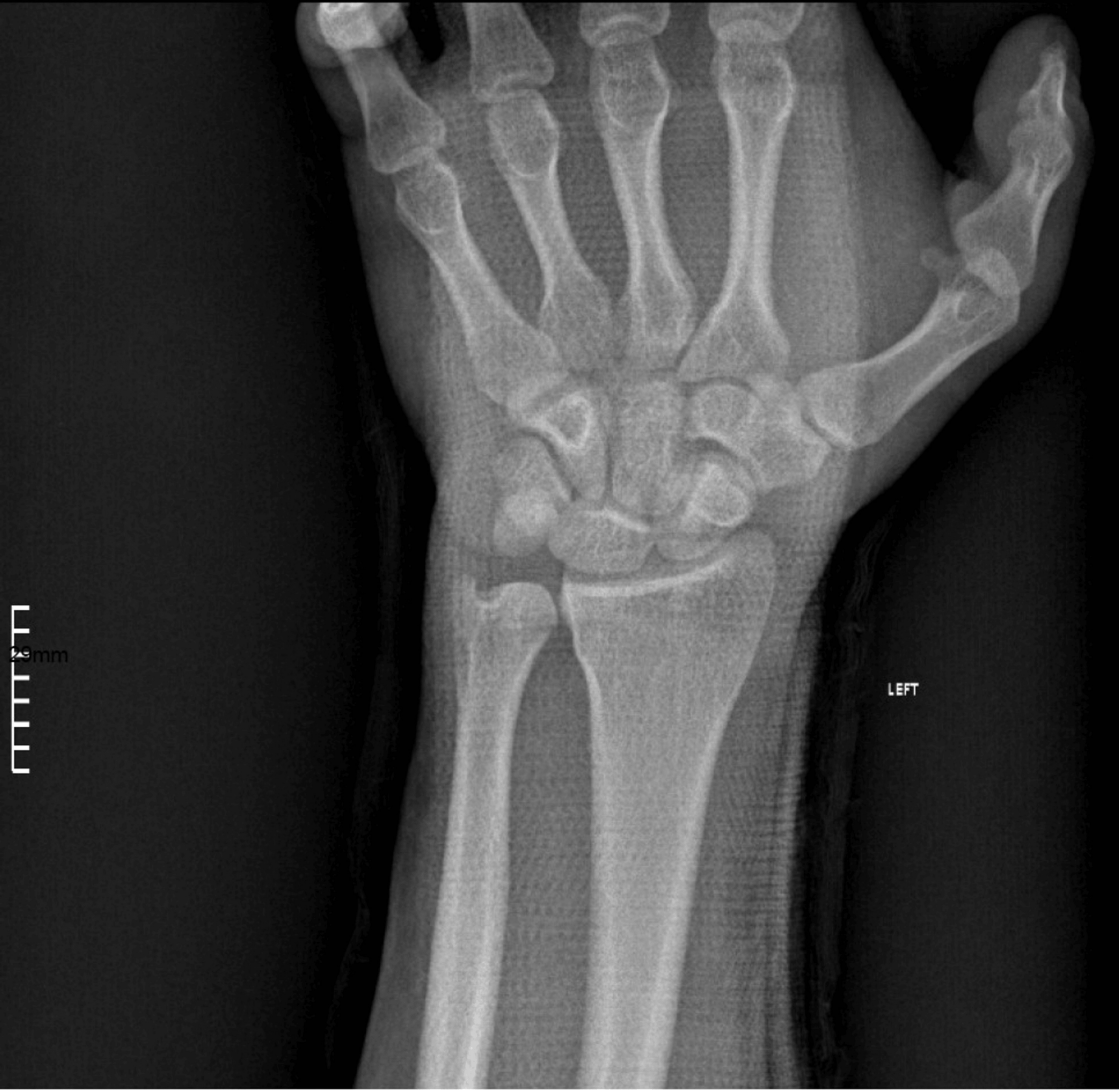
Post-reduction anteroposterior X-ray view of the carpometacarpal joints where the basis is clearly seen
